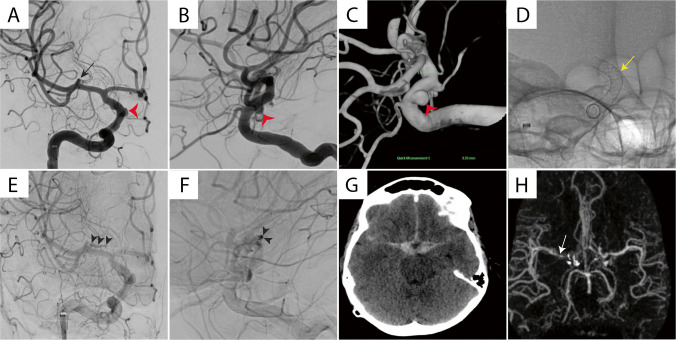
47-year-old female with unruptured right internal carotid artery (ICA) paraclinoid aneurysm treated with Surpass Elite flow diverter complicated by microperforation and subarachnoid hemorrhage. A, B Anteroposterior (AP) and lateral oblique angiographic views of right ICA injection demonstrating a right superior hypophyseal artery aneurysm measuring 2.3 × 2.7 × 2.9 mm with a 3.0 mm neck (red arrowhead). C Rotational 3D reconstruction demonstrating the right ICA aneurysm (red arrowhead). D Magnified unsubtracted radiographic view with Surpass Elite flow diverter deployed, showing good device visualization (yellow arrow). E, F AP and lateral oblique angiographic views of right ICA injection post-Surpass deployment in the early parenchymal phase demonstrating extravascular slow contrast stasis indicative of microperforation around a dominant lateral lenticulostriate artery, which was managed with balloon inflation, heparin reversal and subsequent complete resolution. G Postoperative axial non-contrast head computed tomography demonstrating subarachnoid hemorrhage in the basal cisterns. H Computed tomography angiography reconstruction on postoperative day 7 demonstrating moderate vasospasm in the right M1 segment. The patient was premedicated with dual antiplatelet therapy consisting of aspirin and Brilinta, with P2Y12 reaction units measured at 5, indicating hyperresponder status with excellent platelet inhibition
